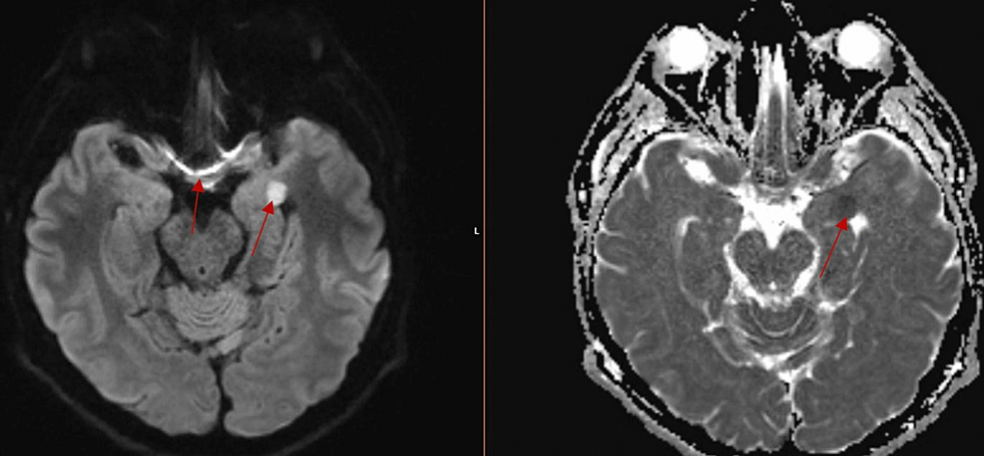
MRI T1/T2 Leptomeningeal enhancement with basilar predominance. Acute infarct in the left temporal lobe.
